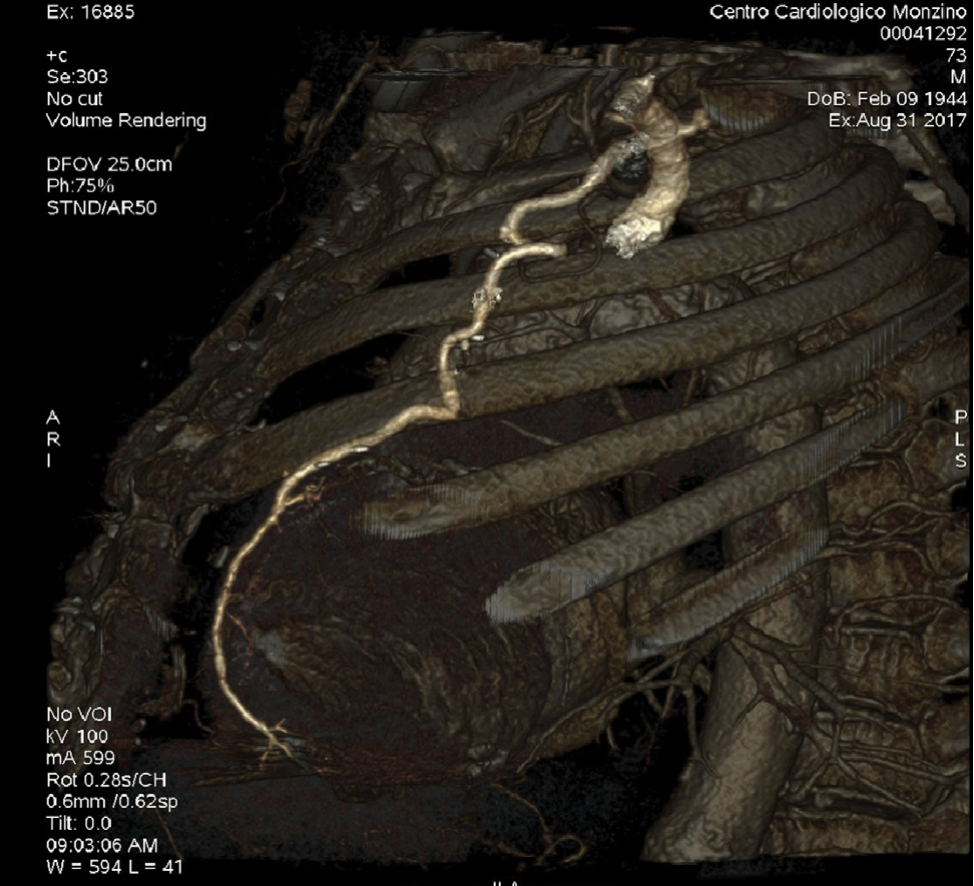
The history of the patient was notable for a previous coronary artery bypass grafting procedure. Computed tomography scan was performed to assess the patency of the anastomosis. In the figure, the left internal mammary artery to anterior descending coronary artery anastomosis is shown.
